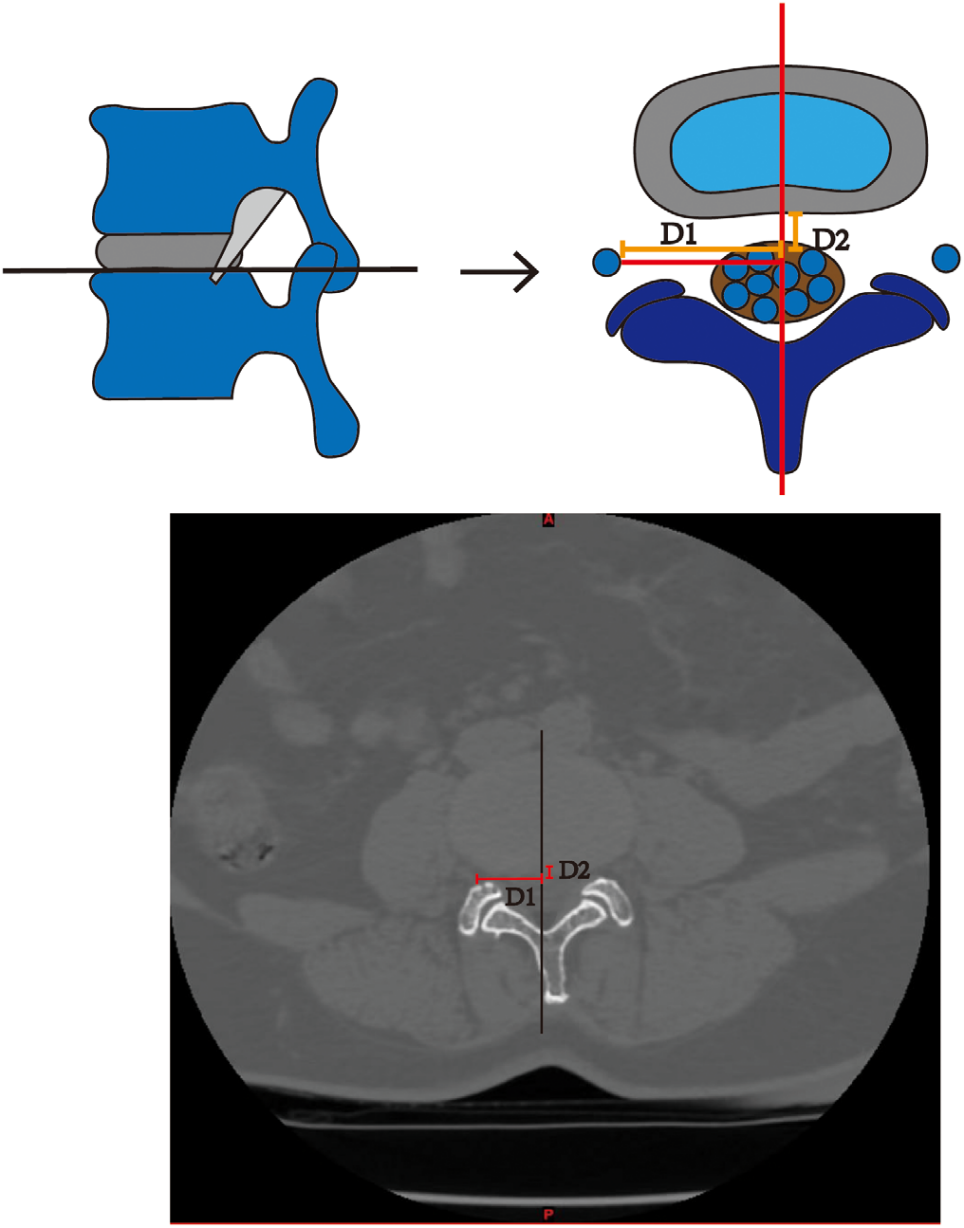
Example of the parameters D1 and D2 on the transverse plane of the computed tomography (CT) at the inferior margins of the target intervertebral disc. D1: Nerve root–disc horizontal distance; D2: Nerve root–disc vertical distance.
